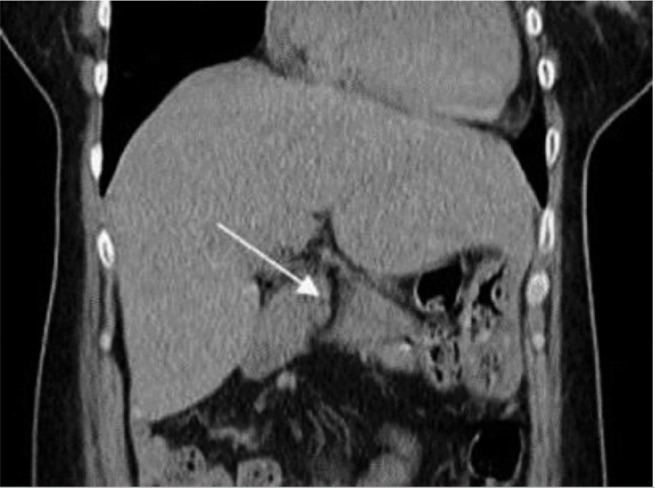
Pancreatic assessment. Coronal CT scan reveals the presence of a hypodense tubular structure that crosses the pancreas longitudinally, with loss of usual morphology with pancreatic tissue on both sides of the duodenum compatible with annular pancreas.
